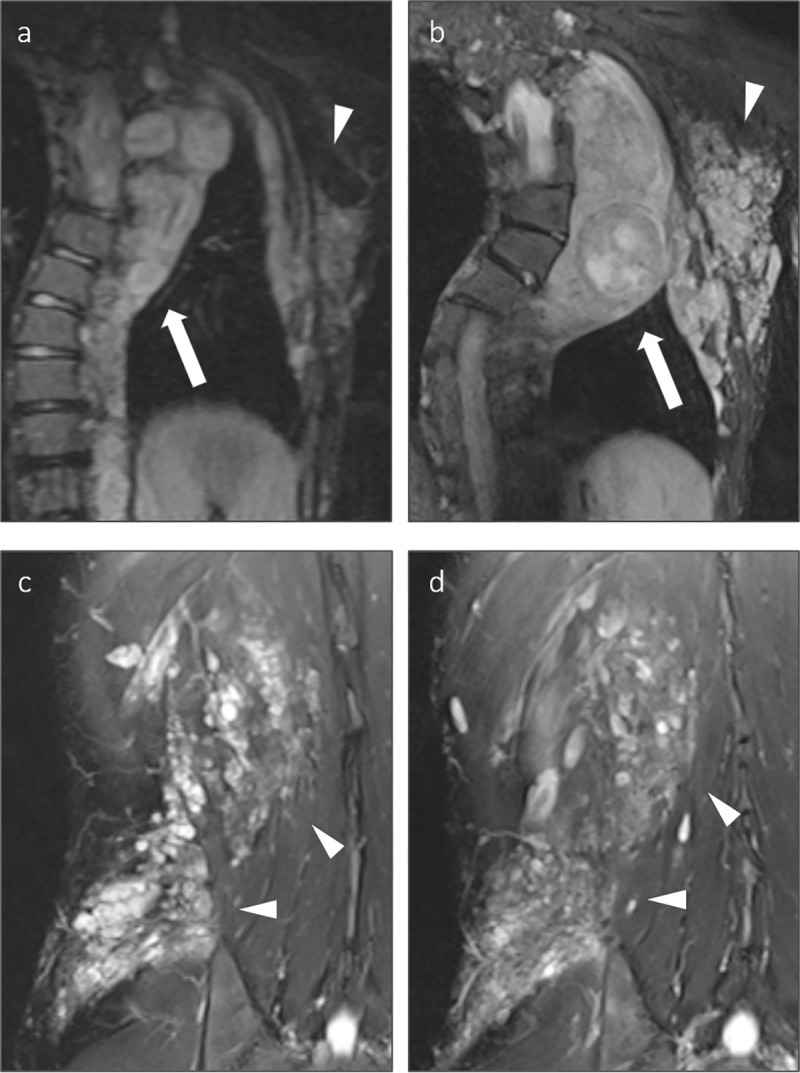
Exemplary presentation of tumor growth in a 12-year-old, type-1 deletion patient (a and b) and a 19-year-old patient with an intragenic mutation of the *NF1* gene (c and d). Displayed are coronal T2-weighted, fat saturated MR images of the thorax (a, b) and the right flank (c, d). The type-1 deletion patient displayed significant growth of a paraspinal plexiform neurofibroma of the thorax with paraspinal and axillary extension, including a distinct nodular lesion within the plexiform neurofibroma (arrow). The interval between the examinations was eight years. In contrast, the patient with an intragenic mutation displayed no significant growth of the plexiform neurofibroma of the right flank (arrowheads) over an interval of five years.
